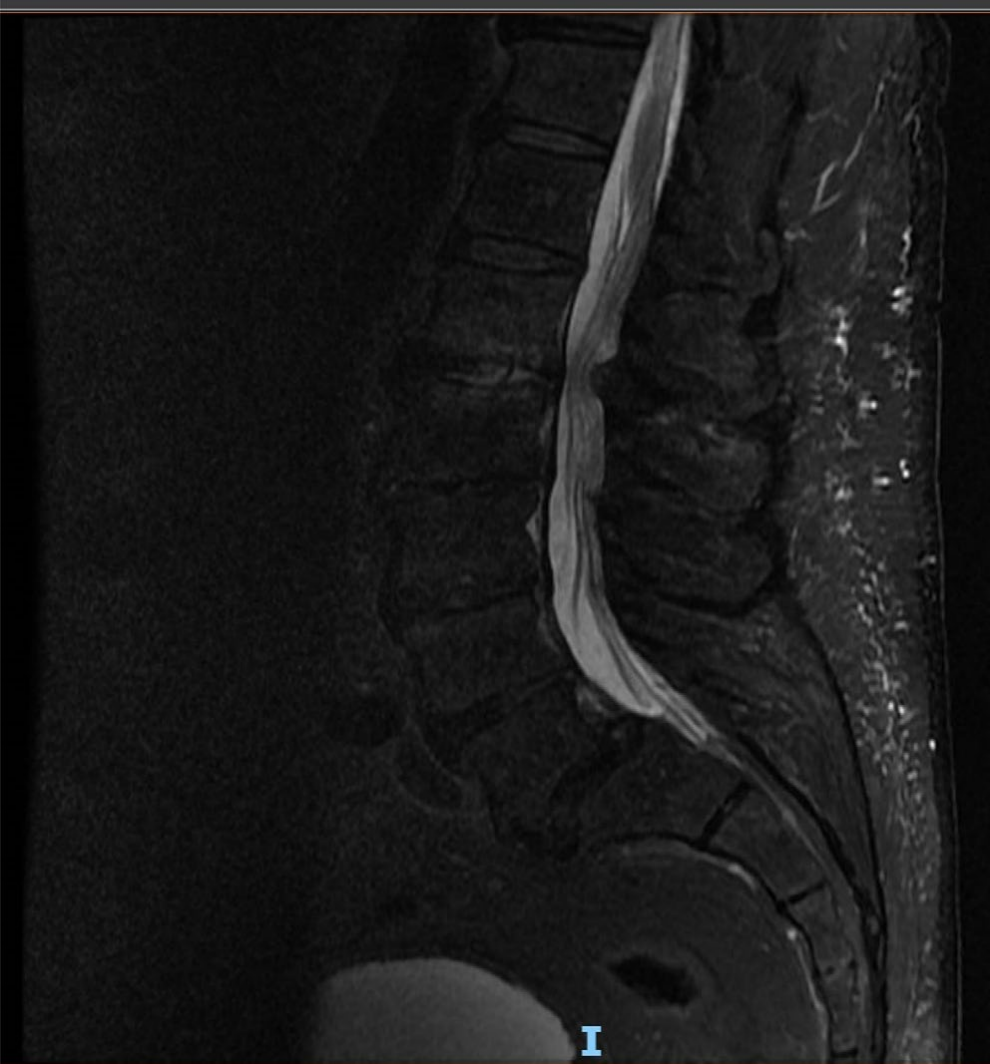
Magnetic resonance imaging of lumbar spine showing degenerative changes
